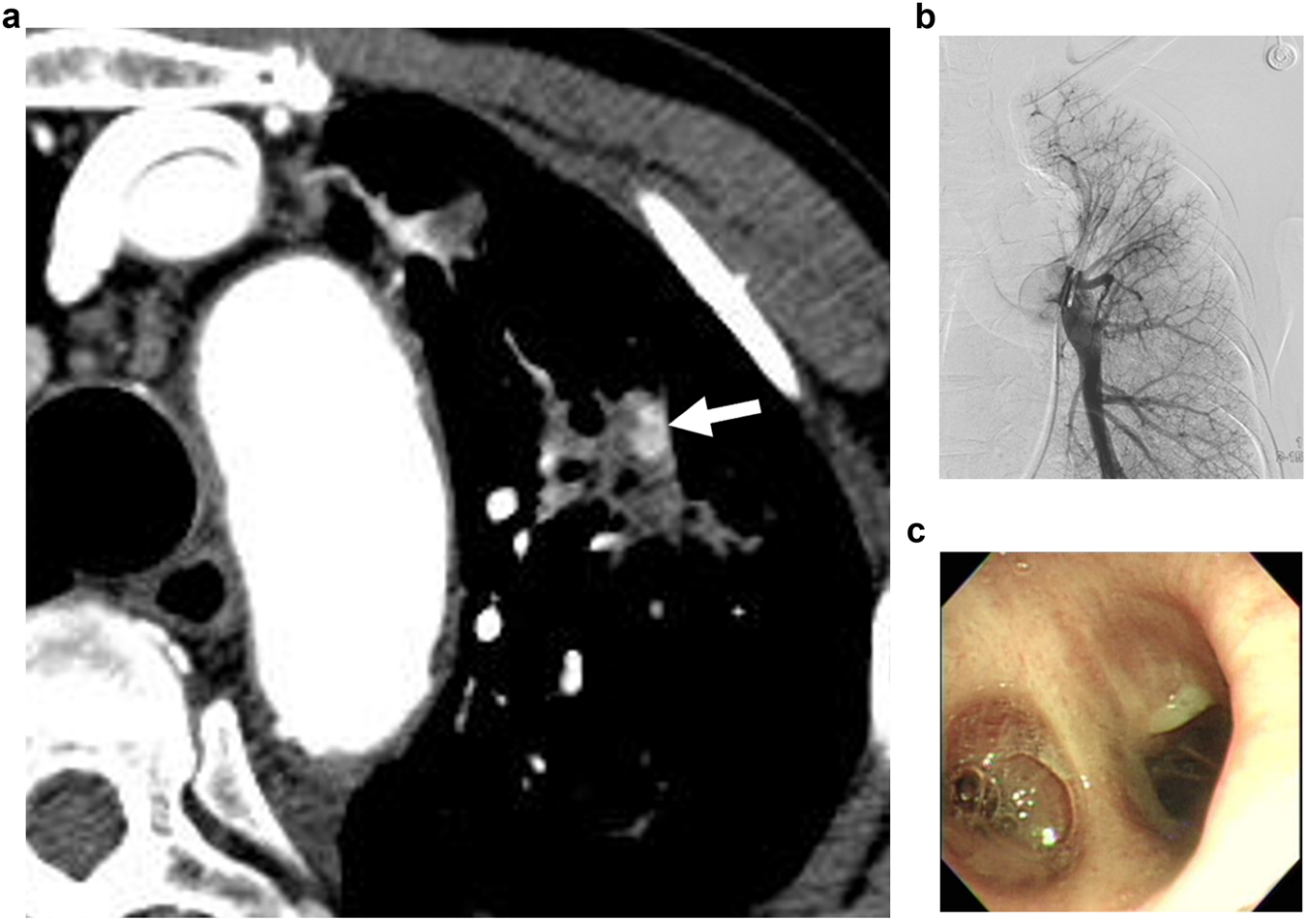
A 72-year-old man with a history of tuberculosis was admitted with blood-tinged sputum. a CT angiography shows a pulmonary artery pseudoaneurysm (PAP) in the left upper lobe of the lung (arrow). b Pulmonary arteriography was performed using a pigtail catheter. However, the PAP could not be visualized. c The patient underwent conservative treatment with tranexamic acid and antitussive administration. After treatment, the patient’s blood-tinged sputum improved. Upon performing a bronchoscopy, no bleeding in the corresponding area was detected
